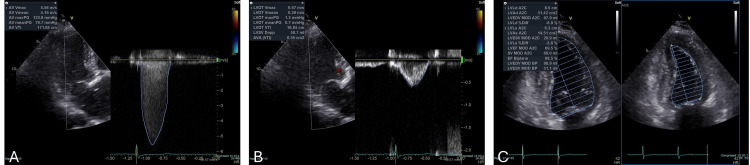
Preoperative transthoracic echocardiography A: Continuous-wave Doppler across the aortic valve showing a peak transvalvular velocity of 5.56 m/s and a peak pressure gradient of 123.8 mmHg.
